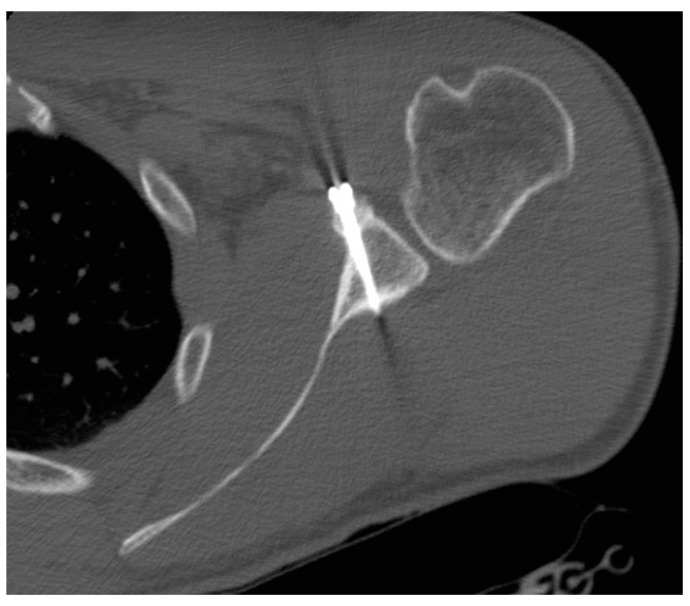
Axial computed tomography scan of a united coracoid graft.
